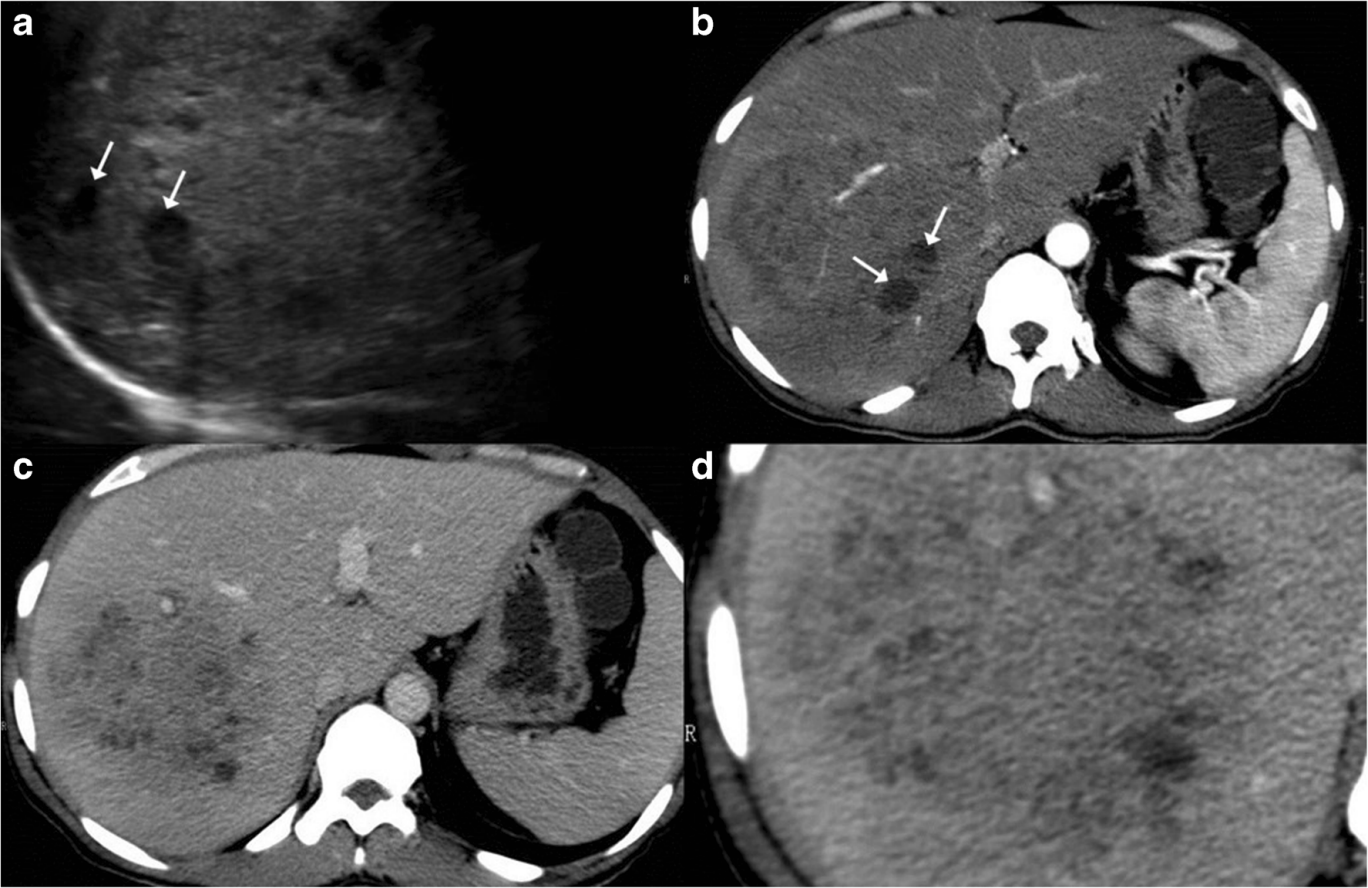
A 27-year-old man with fever, anorexia and isolated hepatic involvement. a Ultrasound shows an ill-defined heterogeneous area in the right lobe with a few well-defined hypoechoic areas (*arrows*) suggestive of liquefaction. b, c Arterial and portal venous phase reveals a well-circumscribed enhancing mass composed of multiple tiny abscesses. Vessels can be seen coursing through the lesion without being attenuated or infiltrated. d Magnified view shows tiny abscesses in a large complex mass giving a ‘cluster’ appearance
